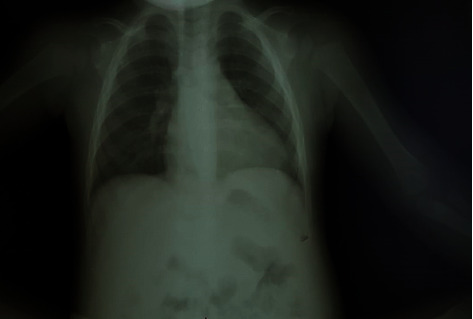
Posteroanterior chest radiograph depicting cardiothoracic ratio >50% by cardiac silhouette.
